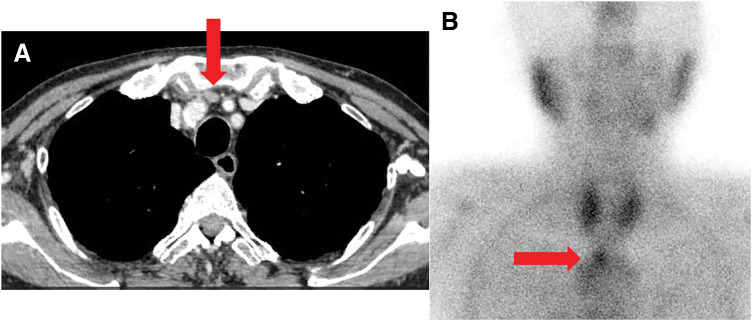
(A) Contrast-enhanced chest CT scan (axial view). A 10-mm well-defined nodule (red arrow) is identified in the superior mediastinum. (B) ^99m^Tc-MIBI scintigraphy. The delayed image reveals a significant nodular accumulation (red arrow) in the superior mediastinal region, consistent with an ectopic parathyroid adenoma. ^99m^Tc-MIBI, ^99m^Tc-methoxyisobutylisonitrile
